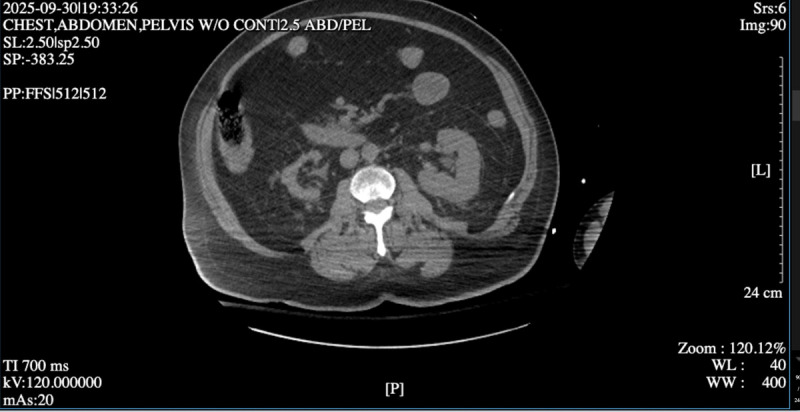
CT scan of the abdomen and pelvis: obstructing 3mm calculus in the proximal left ureter with mild left hydronephrosis; colonic diverticulosis without evidence of diverticulitis
